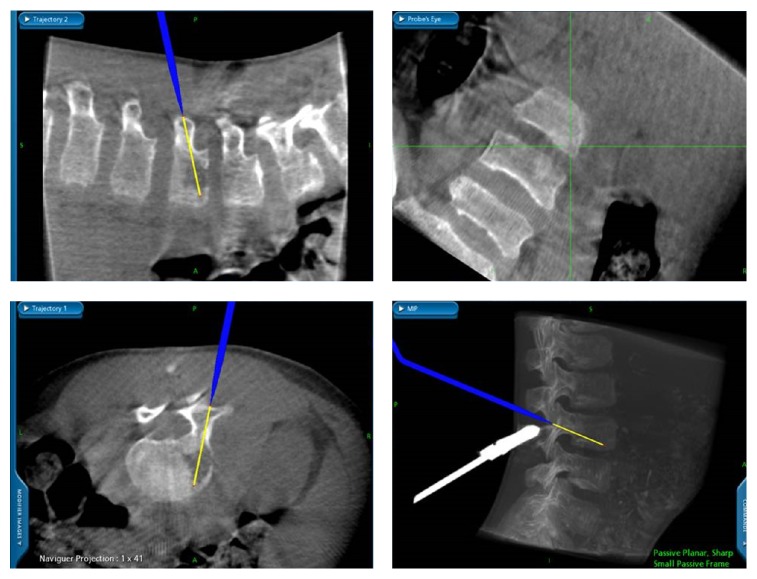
Image during CT-guided aspiration biopsy.
